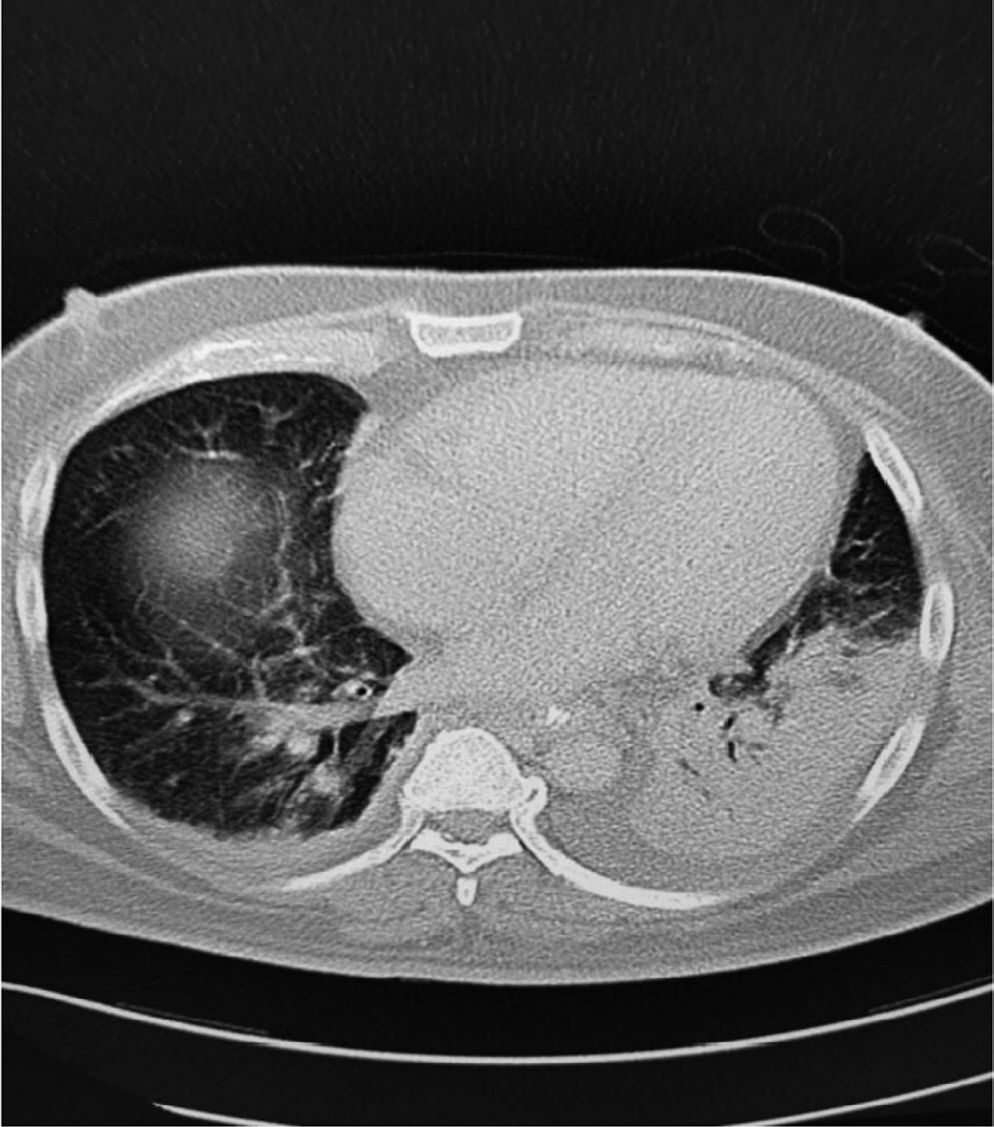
CT scan chest with contrast showed left lower lobe consolidation with air bronchogram
